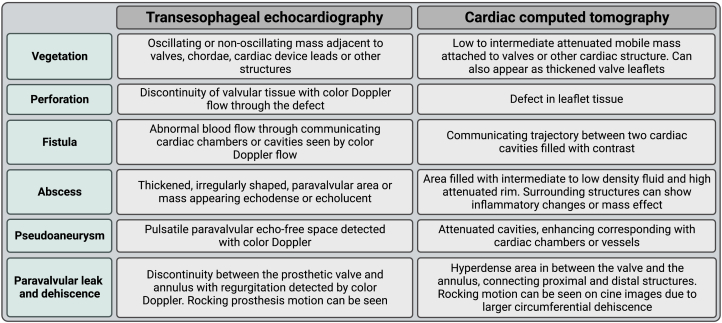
Overview of signs of endocarditis that can be seen on echocardiography and computed tomography.^8^^,^^10^^,^^37^, ^38^, ^39^
